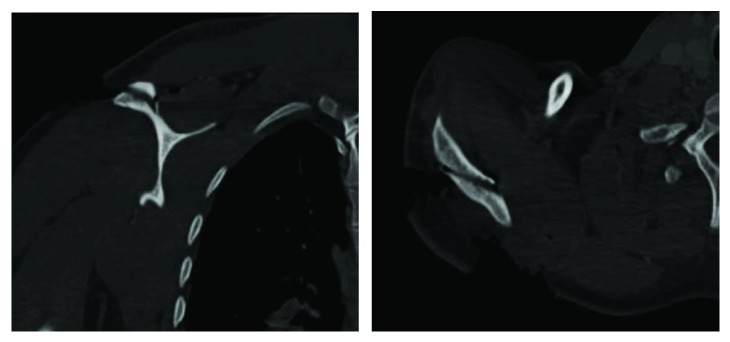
Coronal and axial computed tomography views showing the base of acromion fracture.
